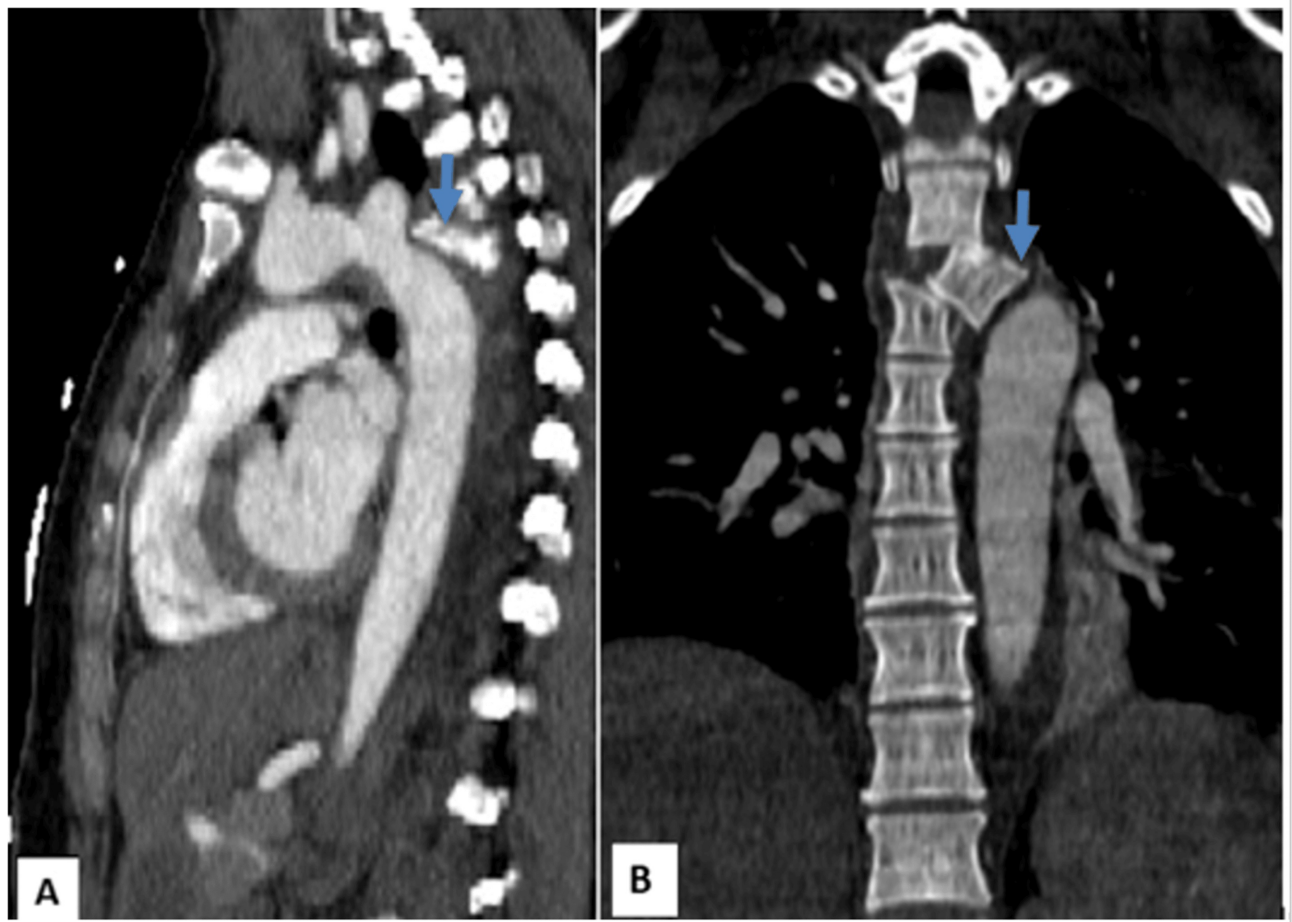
Sagittal (A) and coronal (B) CT angiography Displaced fracture in the left paravertebral region of the fourth dorsal vertebrae (blue arrow), encroaching the descending thoracic aorta although without signs of wall disruption (i.e., absent peri-vascular contrast leakage)
